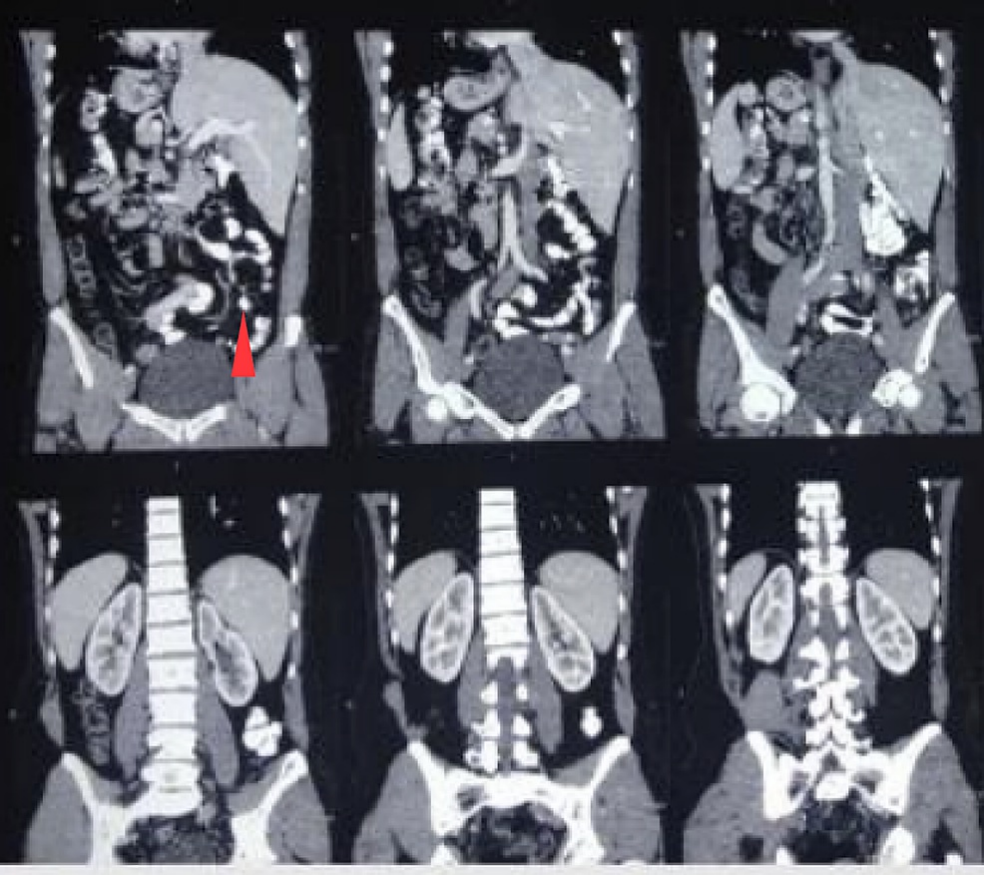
CT of the abdomen confirming SIT and appendicitis (red arrow) SIT, situs inversus totalis
